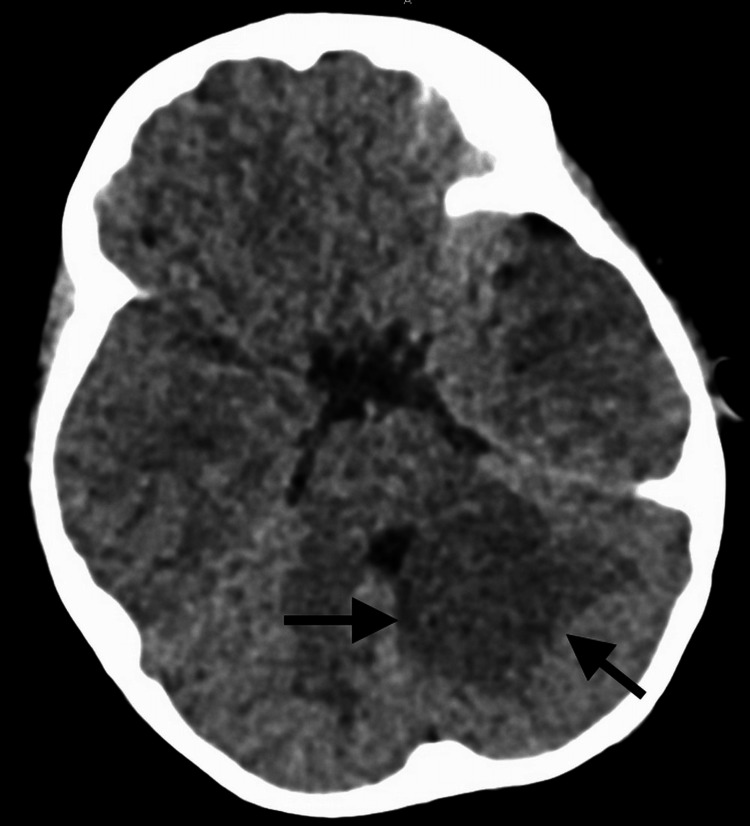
Axial slice of the computed tomography of the head without contrast The arrows note a suspected cerebellar mass.
